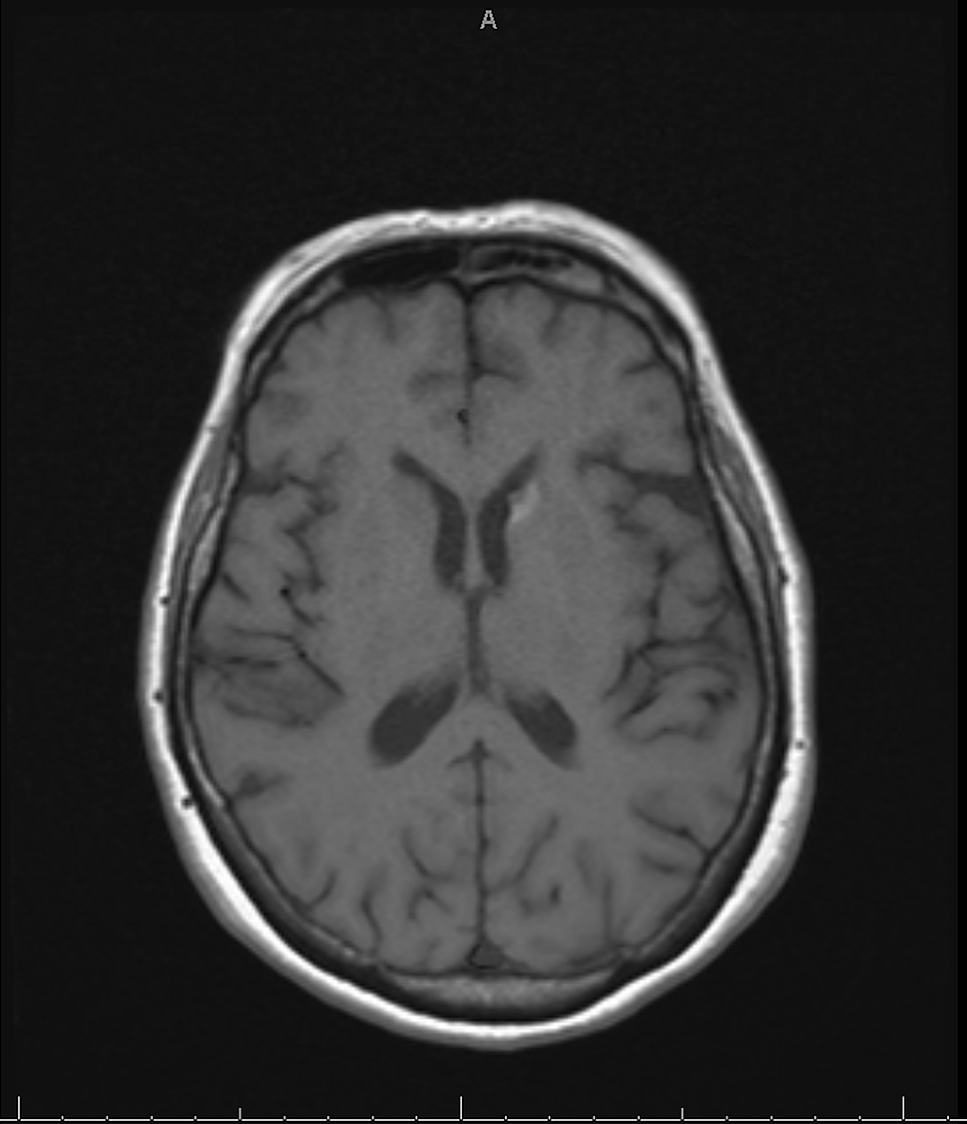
The T1-weighted magnetic resonance image (MRI) shows a reduced mass to 0.7 cm after 2 weeks of therapy for toxoplasmosis
